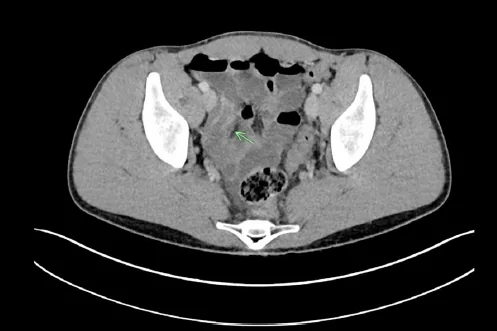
Computed tomography of the abdomen and pelvis demonstrating small bowel wall thickening with associated telescoping lead point consistent with ileoileal intussusception in a patient with IgA vasculitis (arrow).
